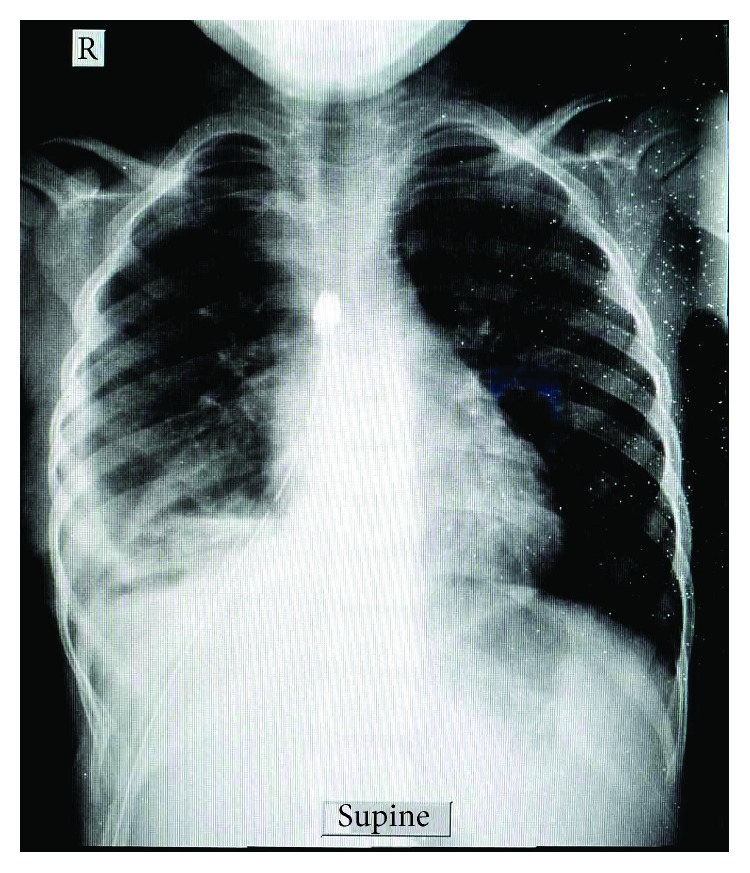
Postoperative chest X-ray with the right thoracostomy tube.
